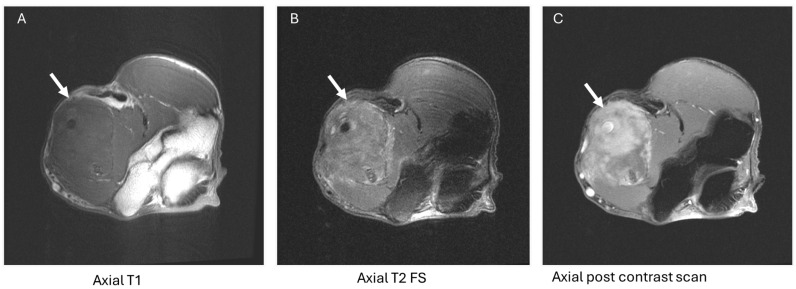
A 63-year-old male with left upper extremity swelling. MRI reveals a T1 hypointense ((A), arrow), T2 hyperintense ((B), arrow) mass with heterogenous enhancement ((C), arrow) encasing the neurovascular bundle within the anterior compartment of the left arm. Mass and bone marrow biopsies confirm the diagnosis of amyloidoma and monoclonal gammopathy.
